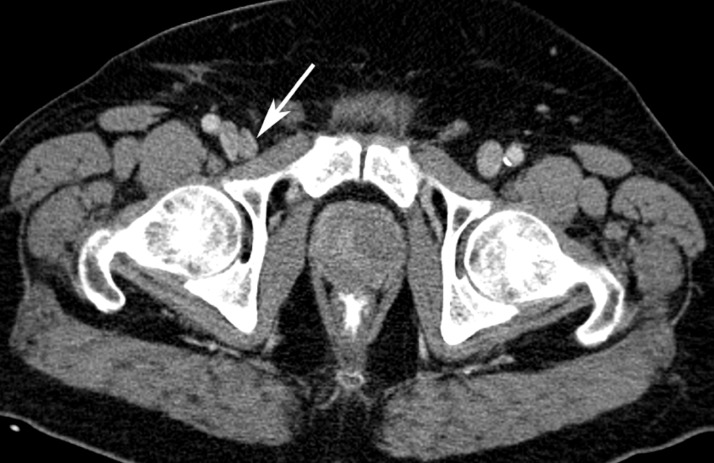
Computed Tomography (CT) Scan of the Pelvis An axial computed tomography image with intravenous contrast through the lower pelvis shows an enlarged right inguinal lymph node (white arrow) adjacent to the right common femoral vein.
